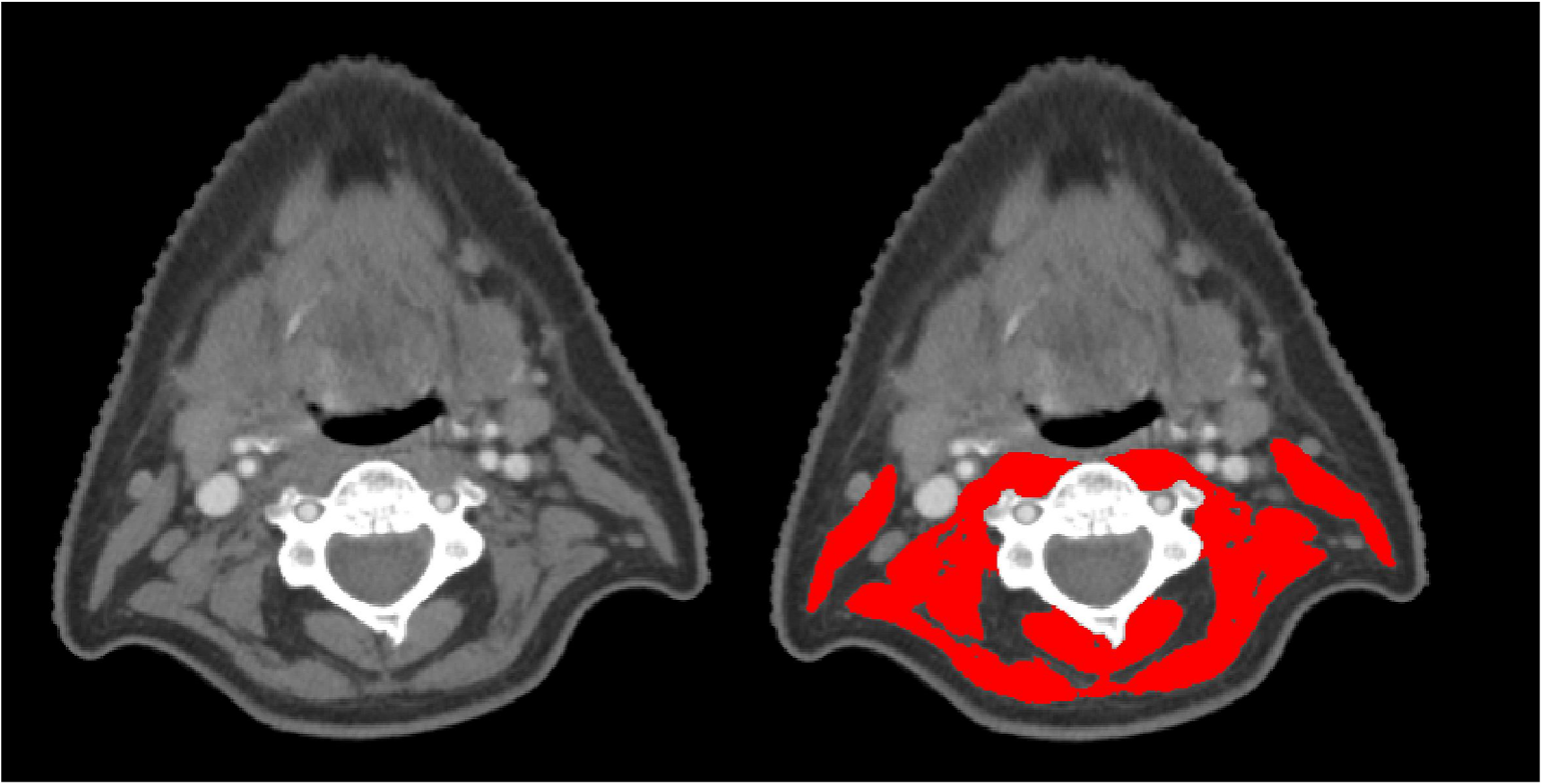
Example of segmentation of skeletal muscle tissue at the level of third cervical vertebra (C3). Two identical axial CT‐slides at the level of C3; the left CT slide shows the skeletal muscle tissue unsegmented, while the right image shows the paravertebral muscles and both sternocleidomastoid muscles segmented in red, using SliceOmatic software. Hounsfield unit (HU) ranges are set at −29 to +150 HU for optimal identification of skeletal muscle tissue [Color figure can be viewed at wileyonlinelibrary.com]
